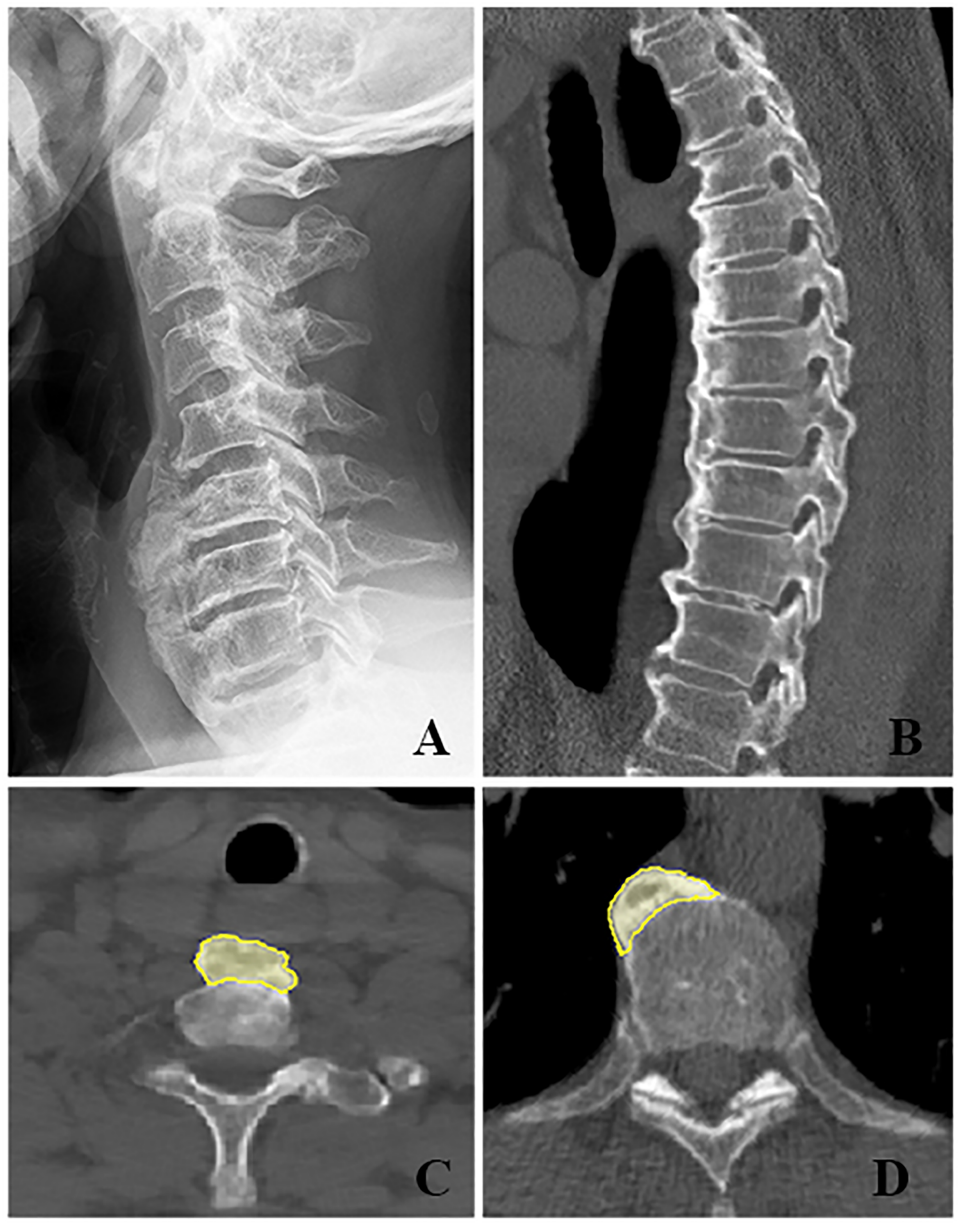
Typical examples of newly formed bone due to DISH in the cervical and thoracic spine. (A) Plain lateral radiograph shows a 69 year old male with DISH in the cervical spine. A solid formation of new bone is extending over at least four vertebral bodies. (B) Computed tomography (CT) visualizes the thoracic spine of a 72 year old male in the sagittal view. The scan shows a flowing ossification of the anterolateral spine with bridging over more than four contiguous vertebral bodies. The intervertebral discs and apophyseal joints are relatively intact in both images. (C + D) The CT scans in axial view demonstrate the differences in position of the new bone formation depending on the region. (C) The CT scan of the cervical spine corresponds to the radiographic image (A) and demonstrates symmetrical hyperostosis (yellow) anterior to the vertebral body and possible displacement of the trachea. (D) The axial CT of the mid thoracic spine in a 58 year old male with DISH shows the newly formed bone on the right anterolateral side with the aorta clearly located on the left anterolateral side.
